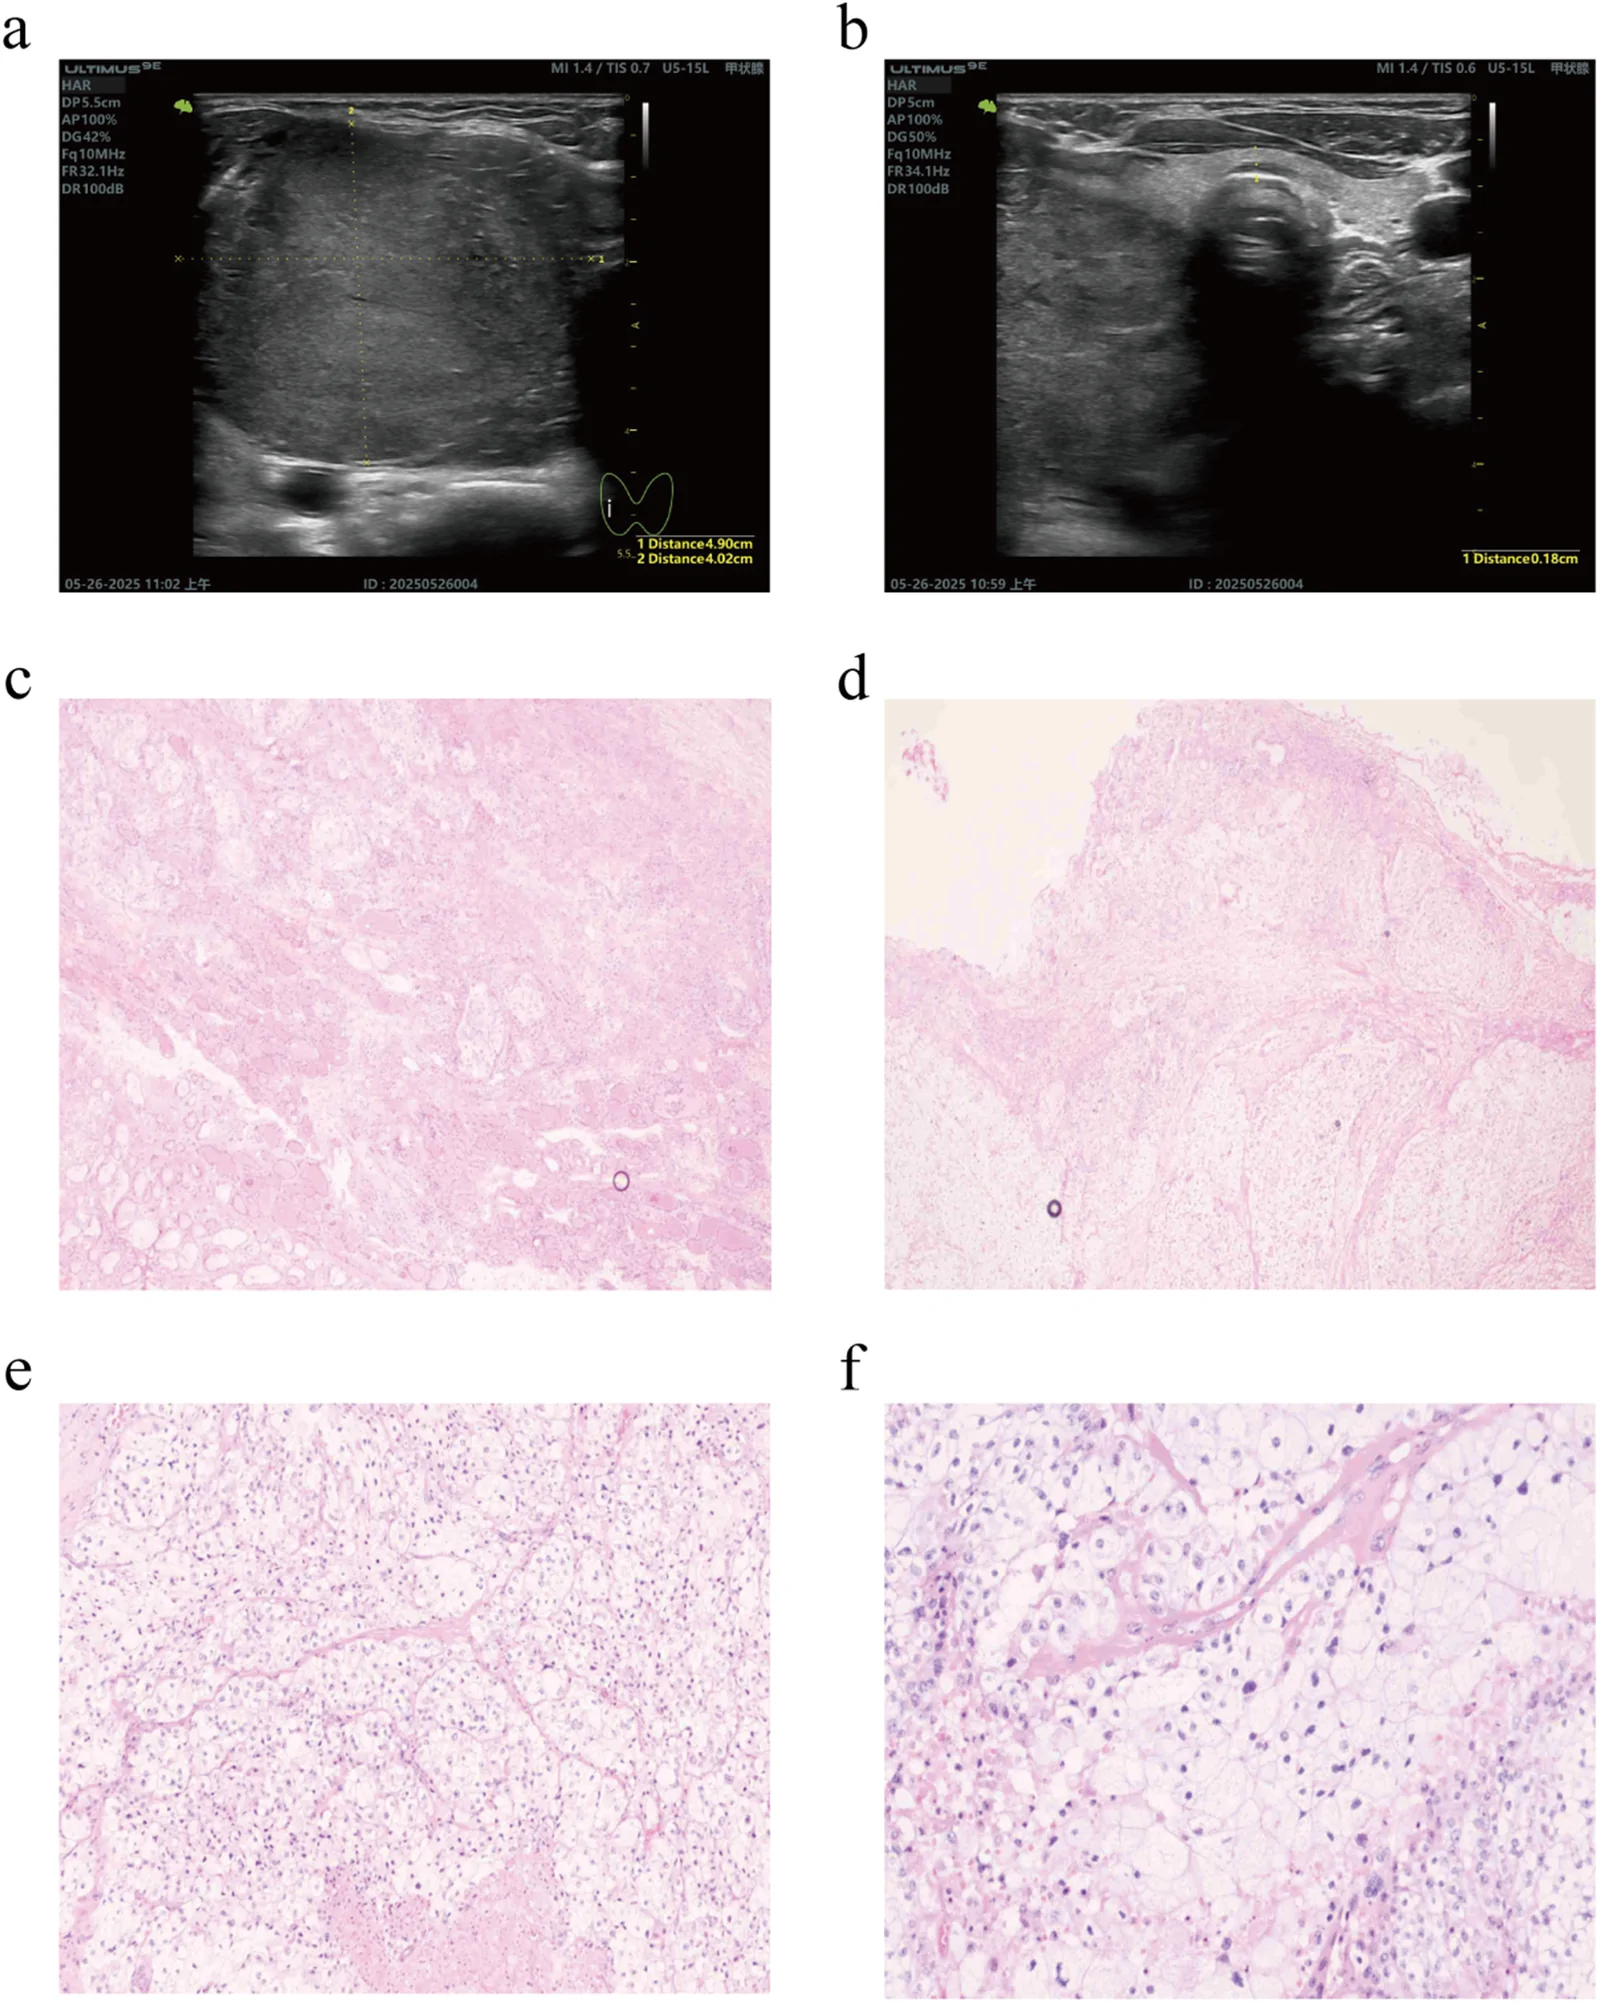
(a,b) Thyroid ultrasound. (c,d) Fast pathologic. (e,f) Pathology.
